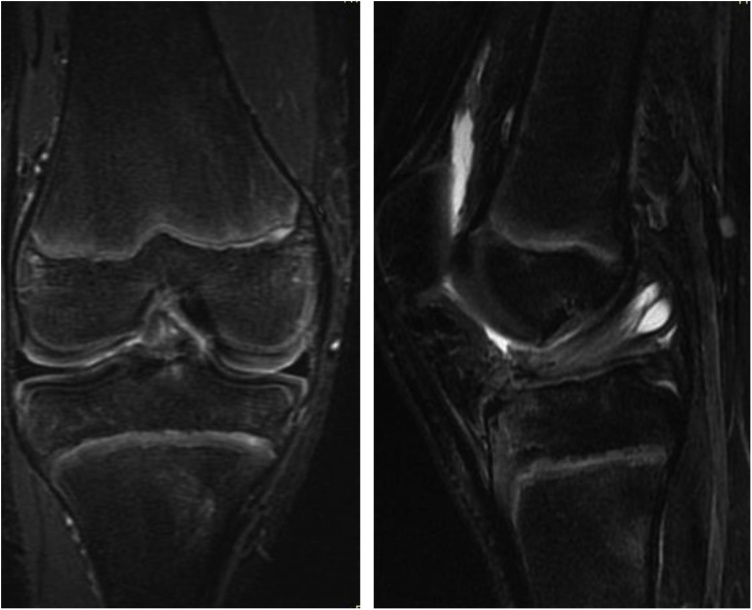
Magnetic resonance imaging obtained 4 months after surgery showed decreased signal intensity around the tibial eminence. Although the signal intensity of ACL fiber was still high, the continuous ACL was confirmed.
